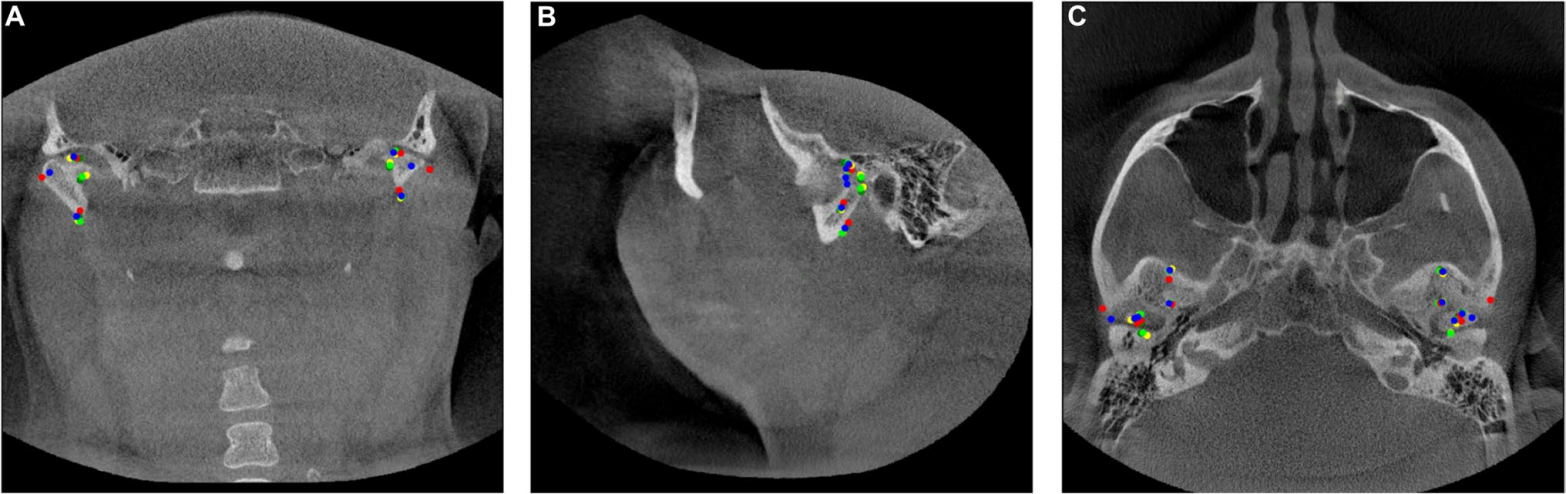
Sample of a CBCT image with paramedian landmarks in coronal (A), sagittal (B), and axial (C) MPR views; red landmarks: GT; lime, green, yellow landmarks: experts 1, 2, 3; blue landmarks: AI
